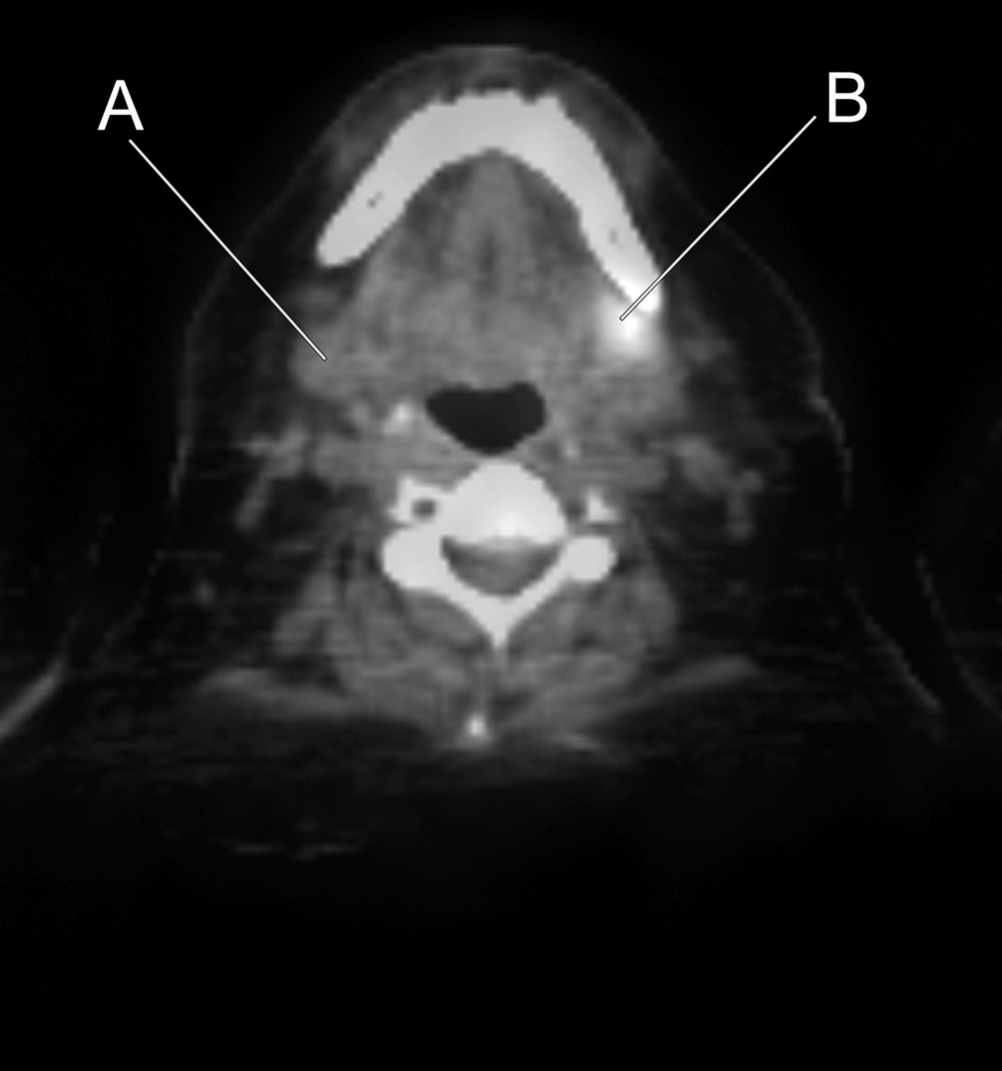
Fused ^18^Fluoro-Deoxy-glucose axial PET CT image demonstrates increased uptake in the left submandibular gland (B). The normal right submandibular gland is shown at this level (A).
